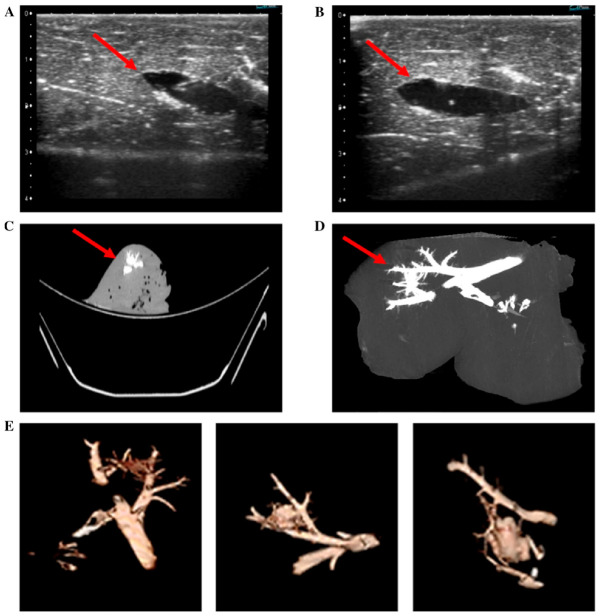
Ex vivo tissue administration of the radiopaque thermo-responsive hydrogel can be visualized using CT and US. Representative (A,B) US and (C,D) CT images of radiopaque hydrogel distribution in ex vivo tissue and (E) CT images of radiopaque hydrogel pooling at parenchymal injection site (arrow), following which, it spreads through vascular and biliary channels and molds to the shape of the liver vasculature via a phase transition [140].
